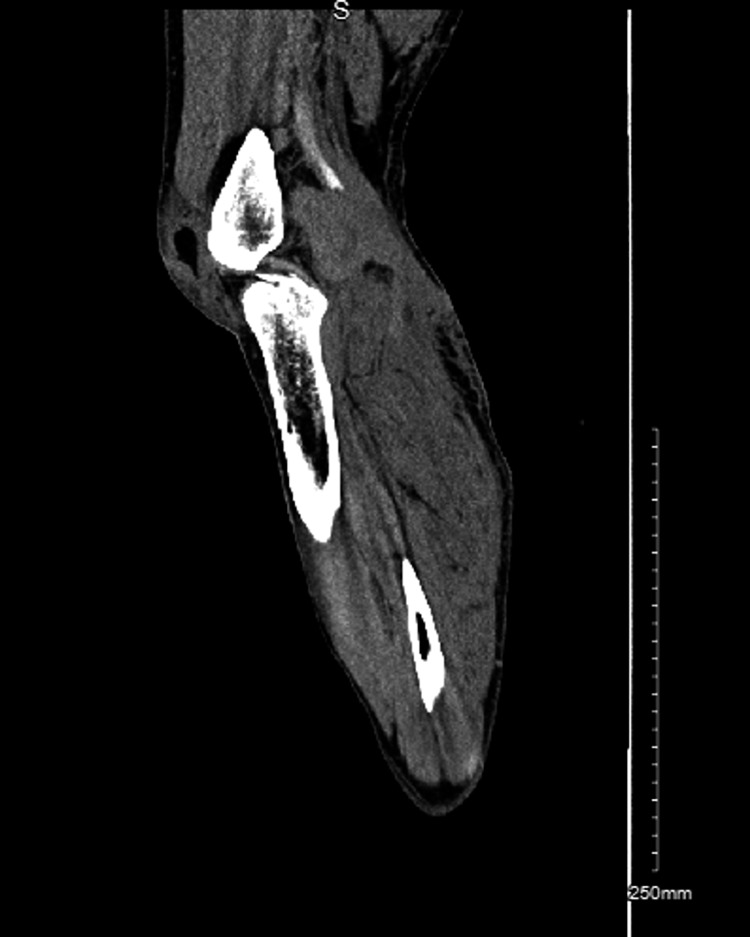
Sagittal view of CT angiography of the left leg showing a large hematoma in the popliteal fossa and abrupt non-opacification of the distal popliteal artery
